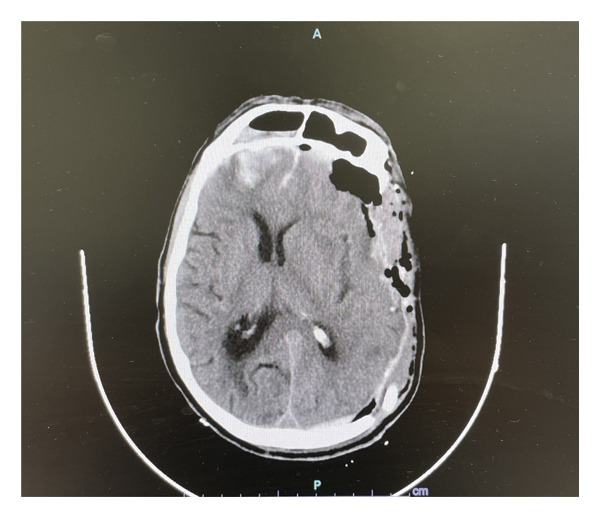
A CT scan after decompressive craniectomy.
